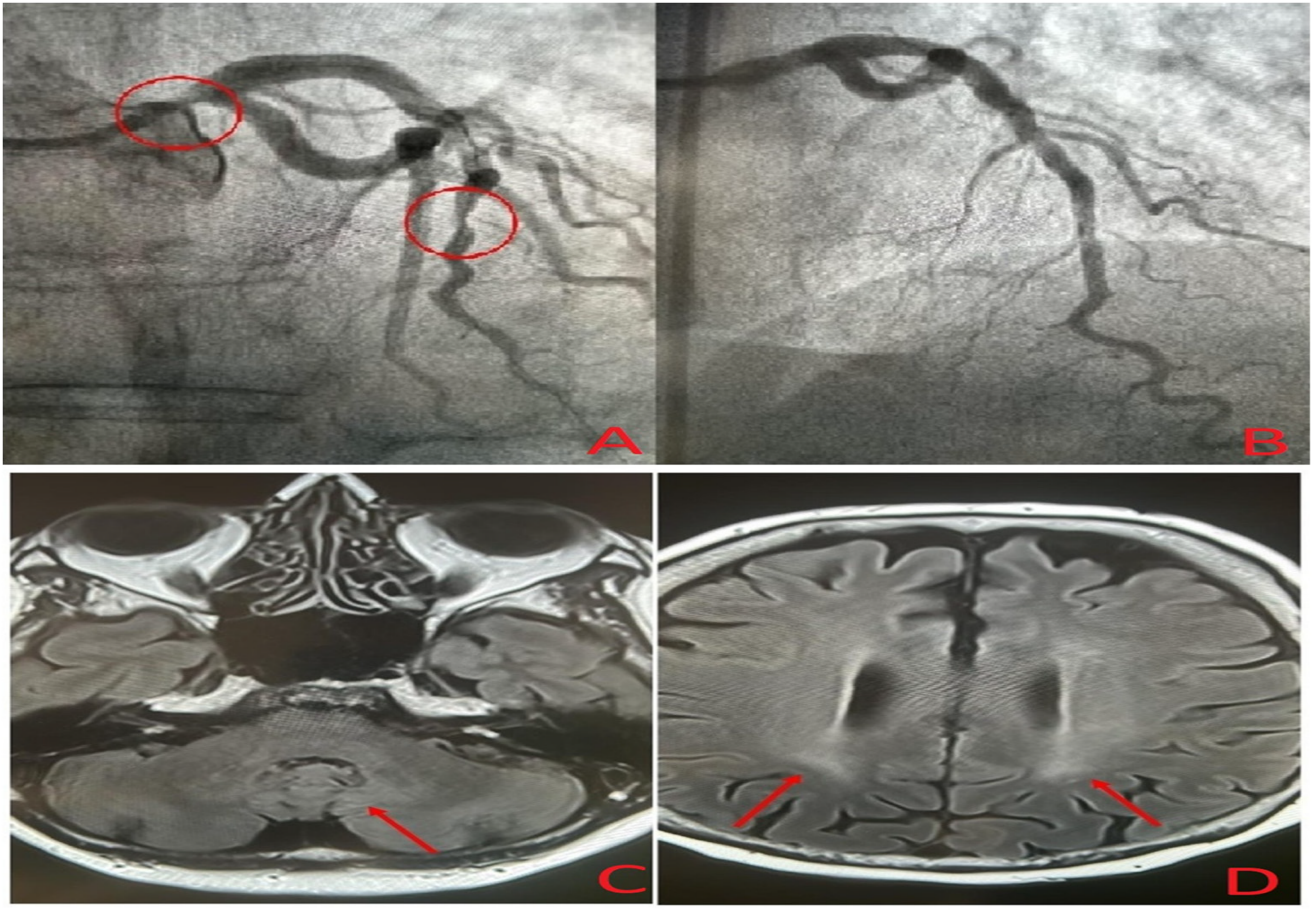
Coronary angiogram showing severe narrowing of the left main and left anterior descending coronary arteries (A), post-treatment coronary angiogram after percutaneous coronary intervention (B), and brain magnetic resonance imaging showing mild symmetrical FLAIR hyperintensities in the dentate nuclei (C) and periventricular white matter (D).
